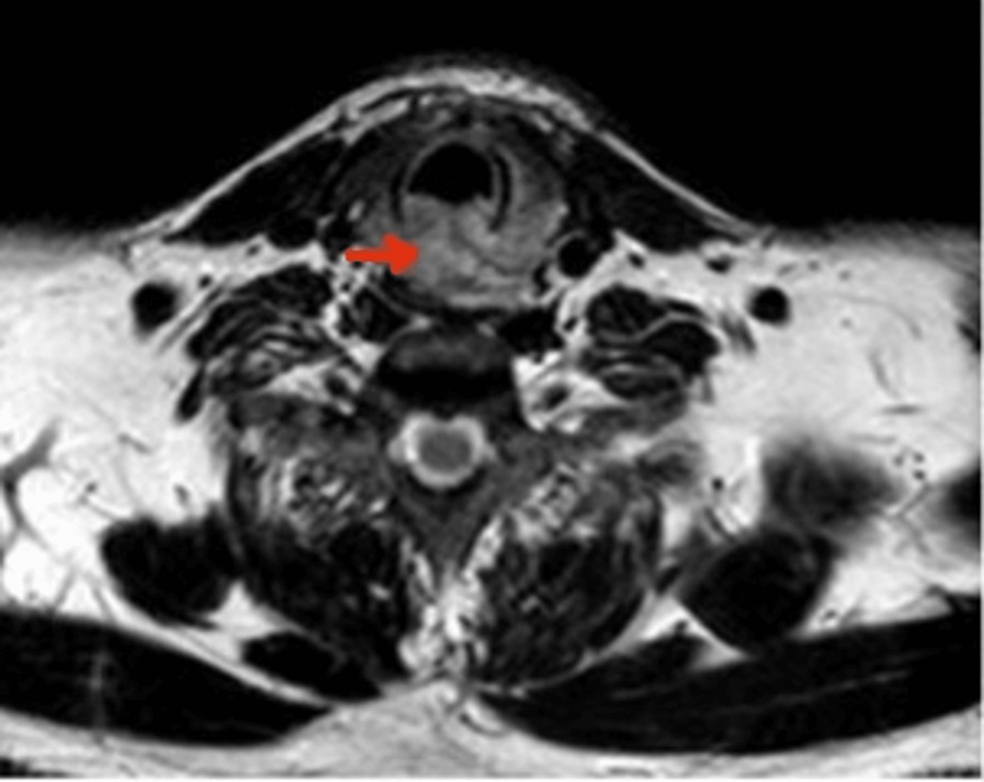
CT axial view showing a posterior endoluminal tumor that invades the posteroinferior perichondrium of the cricoid lamina
